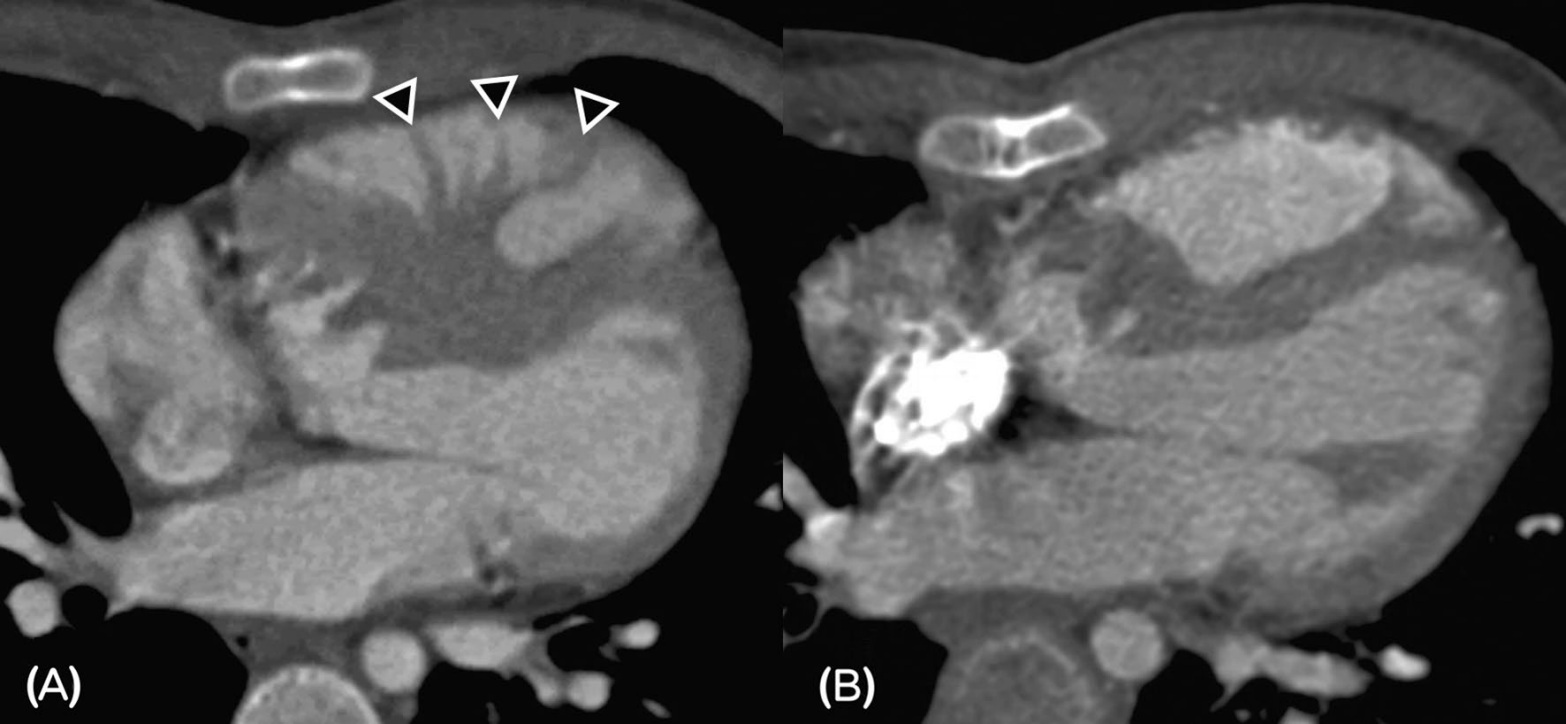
We provide a case of neglected DCRV as an example to emphasize the importance of excluding DCRV before a simple ventricular septal defect (VSD) repair. This patient received VSD repair at birth and had been leading a normal life until he was brought to our hospital due to progressive exertional dyspnea. Initial transthoracic echocardiography yielded increased pulmonary flow and an extremely high RV mid-cavity pressure gradient of over 125 mmHg. Preoperative dynamic cardiac CT raised suspicion for DCRV (right ventricular constrictive ratio = 3.6%) with identifiable muscle bundles crossing the RV cavity inferior to the level of the supraventricular crest with septal hypertrophy (A). The patient received reoperation to remove the muscle bundles and postoperative CT showed no more right ventricular outlet obstruction (B). The patient was completely free of symptoms after surgical resection of the hypertrophic muscles (arrows).
